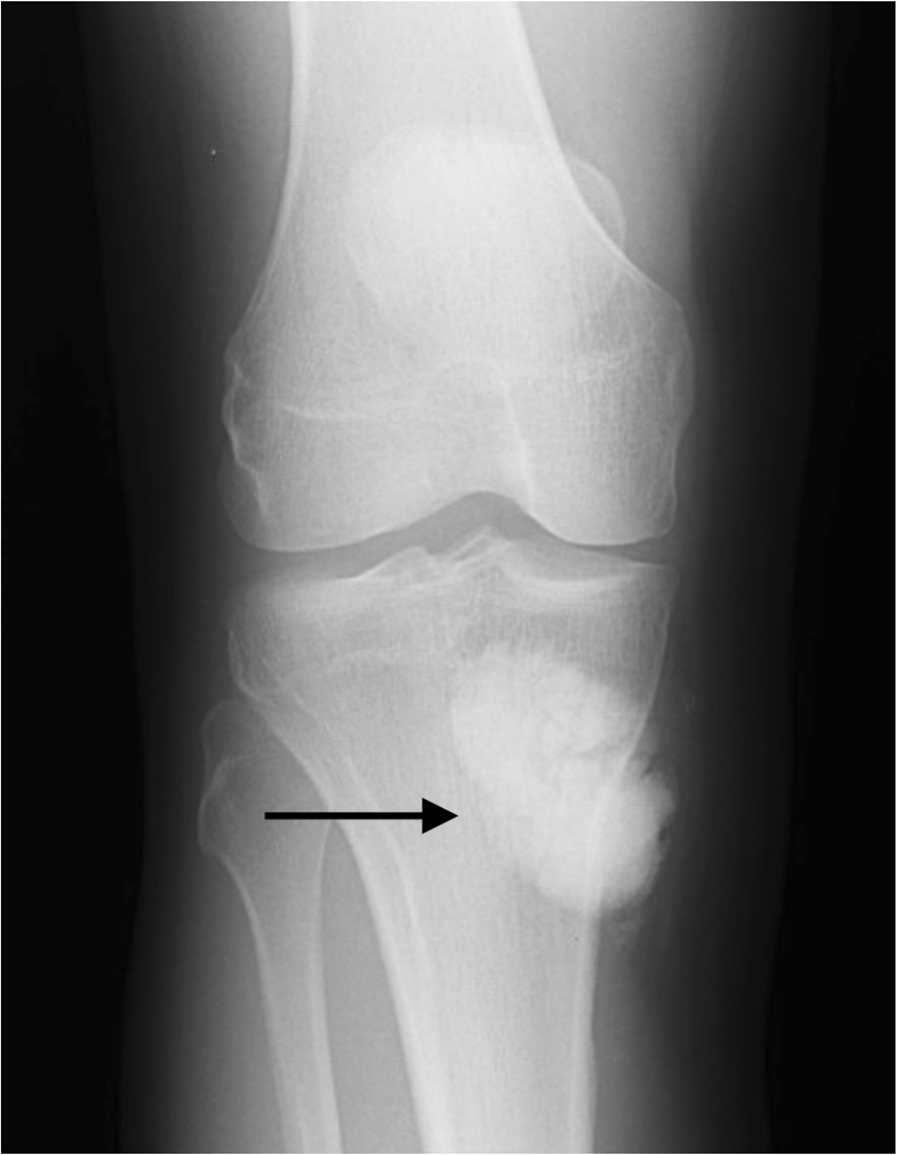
Anterior-posterior plain radiograph of the lesion.
